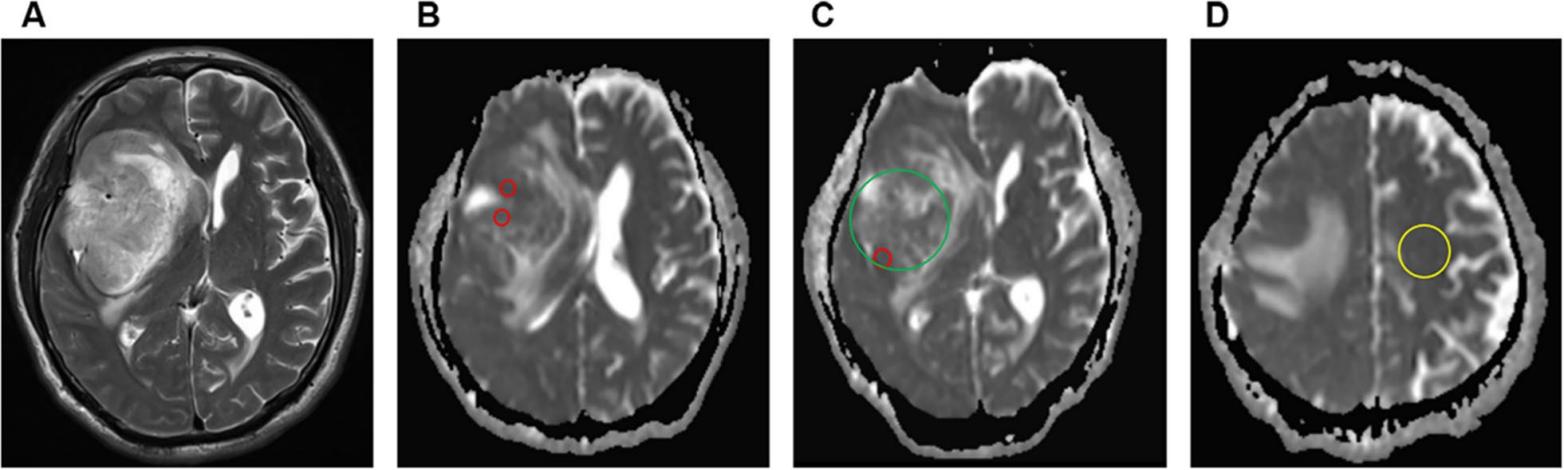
An example of apparent diffusion coefficient (ADC) measurements. A Axial T2-weighted image of a right frontal glioblastoma. B–D ADC maps showing the ROIs used to determine ADC_min_ (three different visually lowest ADC regions, red), ADC_mean_ (largest tumor cross-section measurement, green), and ADC_nawm_ (normal-appearing white matter in contralateral centrum semiovale, yellow)
